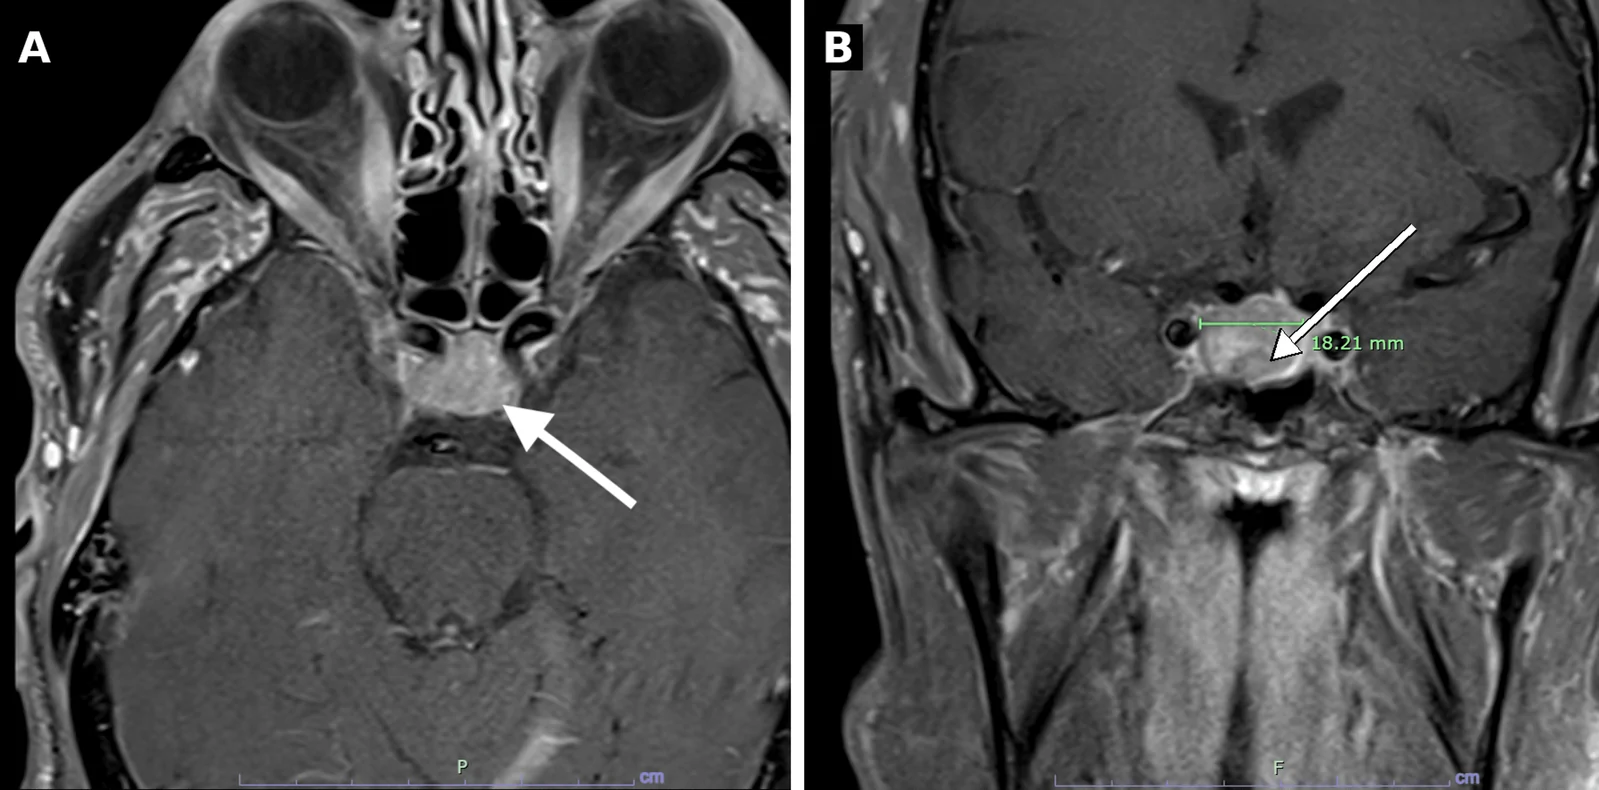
Baseline Preoperative Pituitary MRI Demonstrating Sellar Macroadenoma Initial preoperative pituitary MRI obtained in April 2024. (A) Axial T1-weighted post-contrast image with fat saturation demonstrating a heterogeneously enhancing sellar macroadenoma (arrow). (B) Coronal T1-weighted post-contrast image with fat saturation showing the lesion (arrow) with a transverse diameter of approximately 1.8 cm; residual normal pituitary tissue is compressed and displaced to the left.
